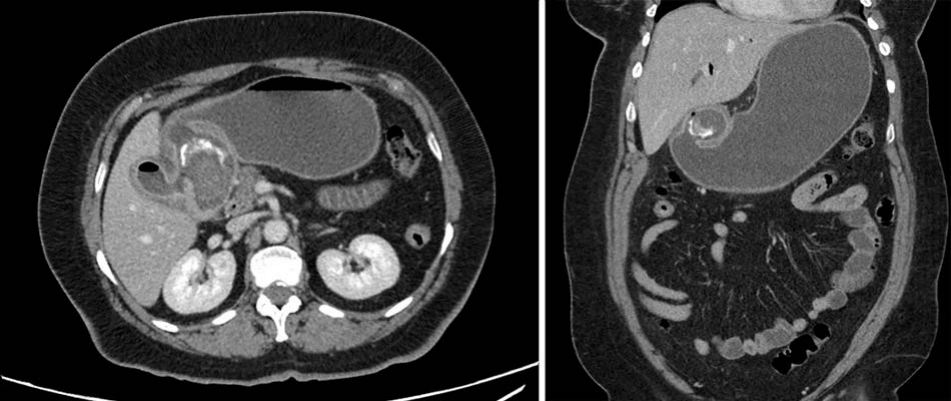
Contrast-enhanced computed tomography (CT). Axial and coronal views of a 2.6 cm stone in the proximal duodenum, gallbladder wall thickening, fat stranding in the porta hepatis and pneumobilia in the non-operative patient.
